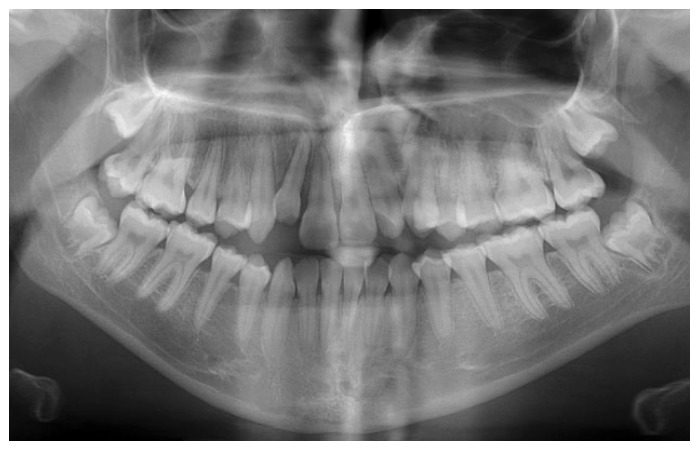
Panoramic radiograph after traumatic injury.
